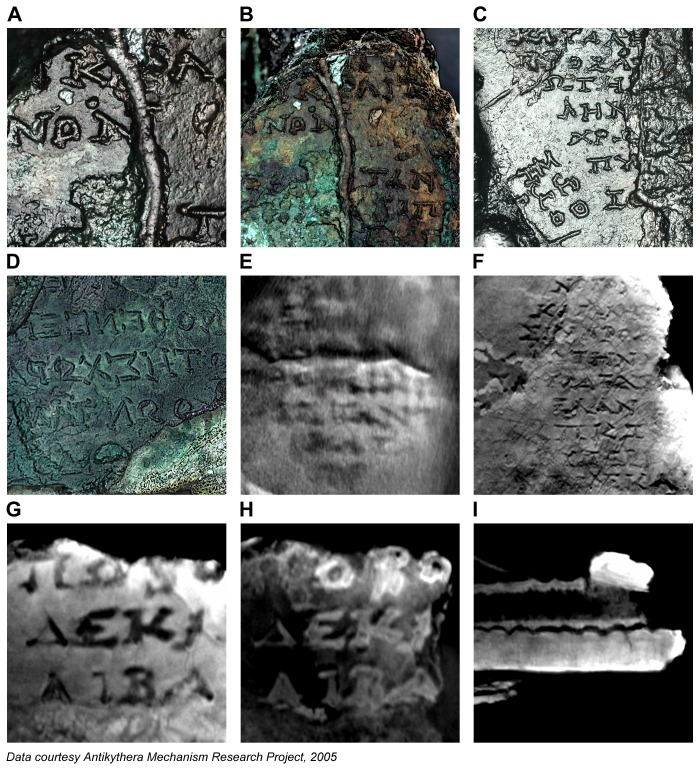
Inscriptions data from the back plate. (A) Fragment A, PTM of back plate with specular enhancement. (B) Fragment A, PTM of back plate with diffuse gain. (C) Fragment A, PTM of back plate with specular enhancement. (D) Fragment A, PTM of impression of back cover with luminance unsharp masking. (E) Fragment A, X-ray CT slice of back plate. (F) Fragment F, X-ray CT slice of back plate. (G) Fragment E, X-ray CT slice of back plate. (H) Fragment E, X-ray CT slice of accretion layer. (I) Fragment E, orthogonal X-ray CT slice of back plate and accretion layer.
